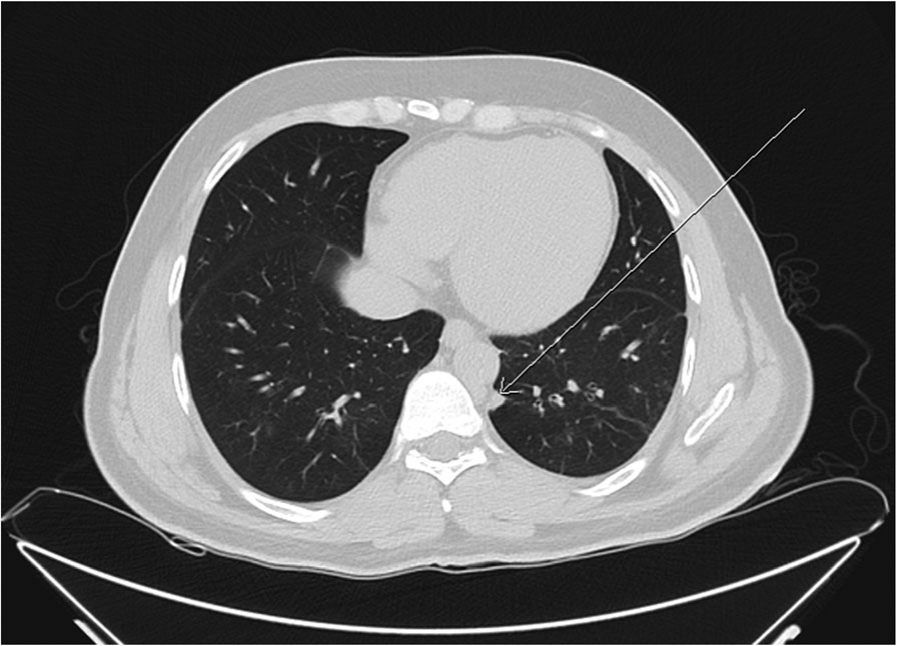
Pre-operative chest CT scan showing the location of pulmonary nodule in patient 3. Preoperative chest CT scan – axial view – pulmonary window, showing a left lower lobe pulmonary nodule as indicated by the white arrow
